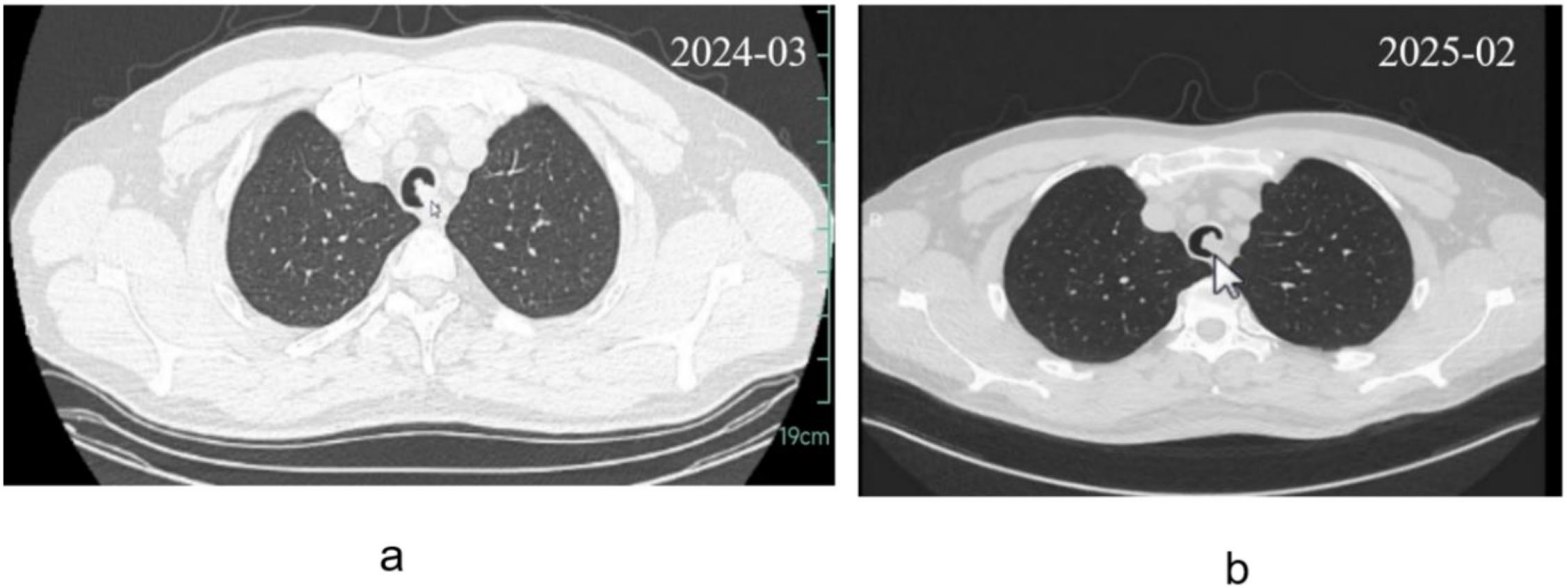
Chest CT shows the tracheal mass: (a) Initial imaging of the tracheal mass; (b) Follow-up imaging one year after discovering a tracheal mass.
